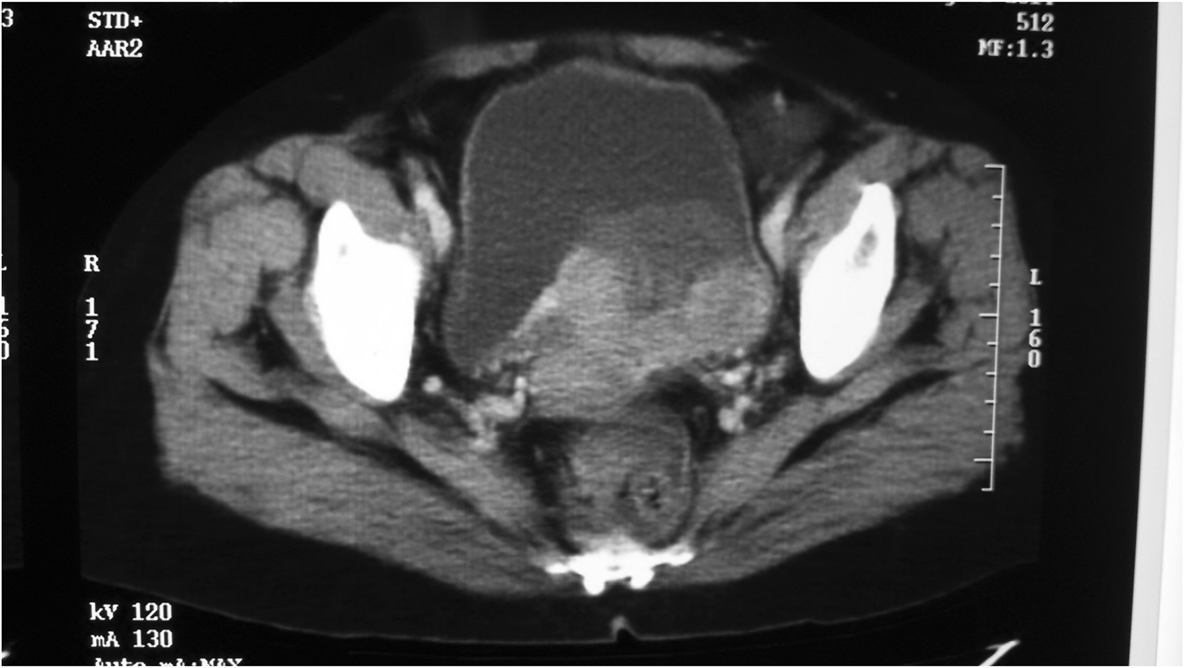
CT scan showing a large irregular mucosal lesion in the left base of the bladder
